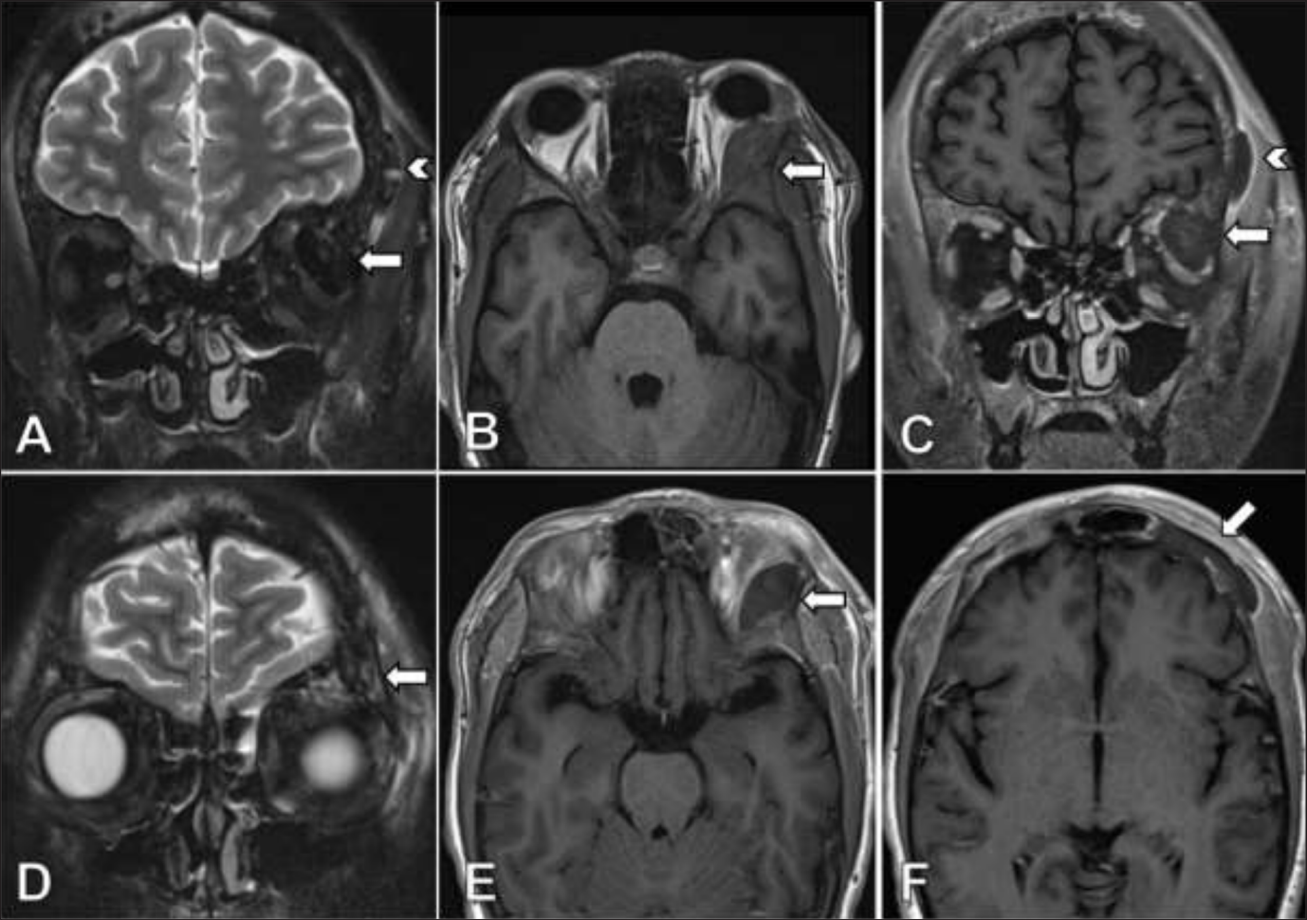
Coronal TSE T2-weighted image with fat suppression (A) shows a markedly hypointense lesion located in the lateral aspect of the left orbital roof (arrow) and a second lesion lining the external table of the frontal bone on the left side (arrow head). On axial TSE T1-weighted images (B) the lesion exhibits a isointense signal compared to the adjacent bone, without lesional enhancement after injection of gadolinium (E). Coronal contrast-enhanced TSE T1-weighted image (C) reveals a faint perilesional enhancement, more prominently in the lesion lining the frontal bone on the left (arrow head). Coronal TSE T2-weighted image with fat suppression (D) shows asymmetrical, discrete bone edema and prominent nonenhancing lesions on contrast-enhanced TSE T1-weighted image (F) in the frontal bone on the left side compared to the right side.
